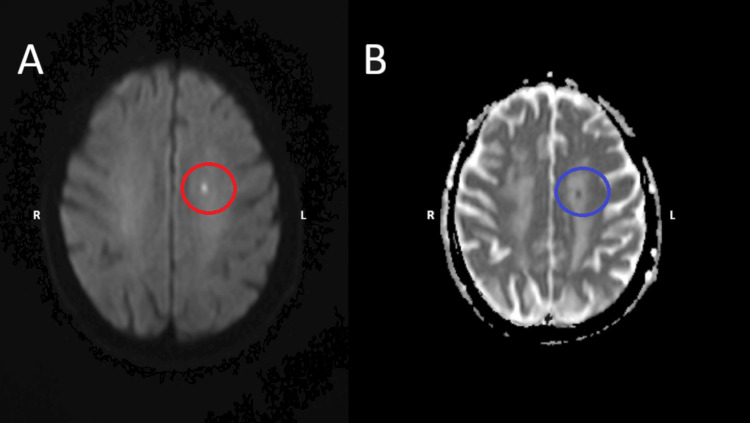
MRI images confirming the lesion Axial MRI brain images demonstrating a focal area of diffusion restriction in the left centrum semiovale, consistent with an acute lacunar infarct.  (A) The DWI image (lesion encircled in red). (B) The ADC image (lesion encircled in blue). DWI: diffusion-weighted imaging, ADC: apparent diffusion coefficient The images have been marked with right (R) and left (L) for orientation.
